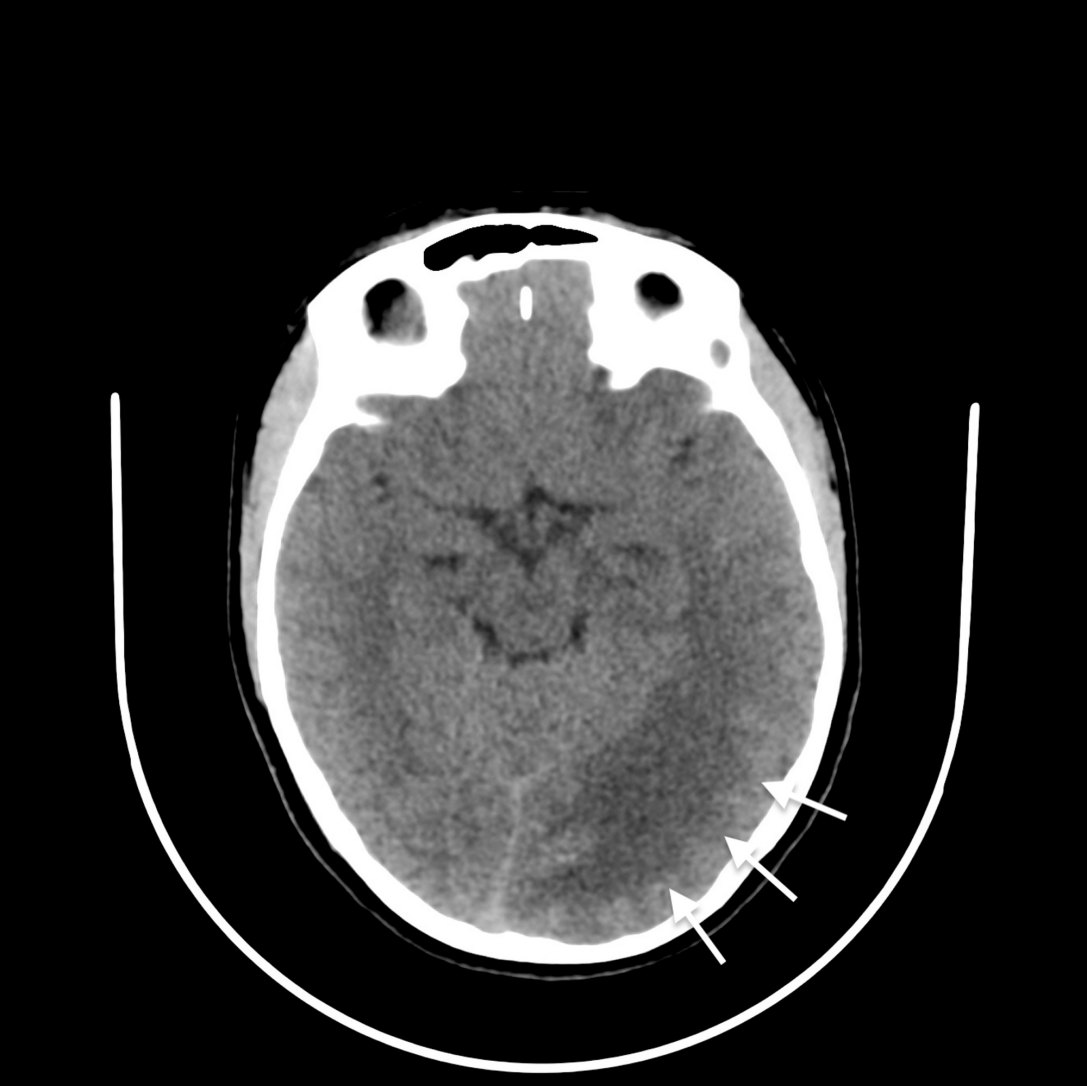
Non-contrast enhanced computed tomography of the head Enlarged hypodense left parieto-occipital lobe with a “mass-like” effect (white arrows).
